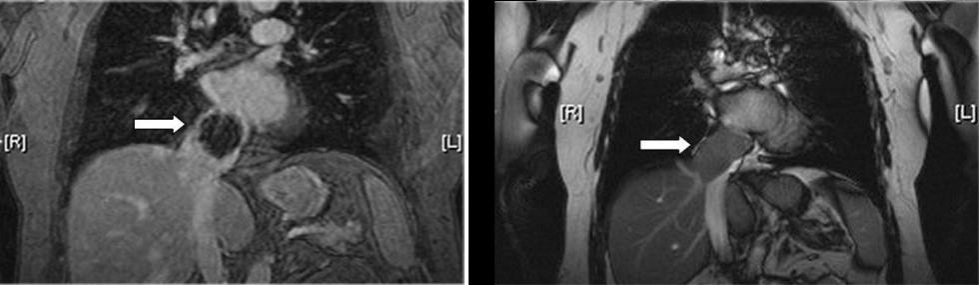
MRA/MRI of the mass in the inferior vena cava. On the left (a), MRA highlighting the tumor mass in the inferior vena cava. On the right (b), MRI highlighting the tumor mass in the inferior vena cava.
